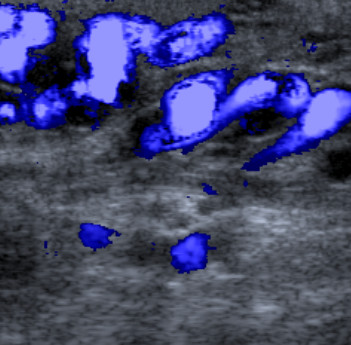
Colour Doppler ultrasound image – variceal gonadal vein in left inguinal canal.
